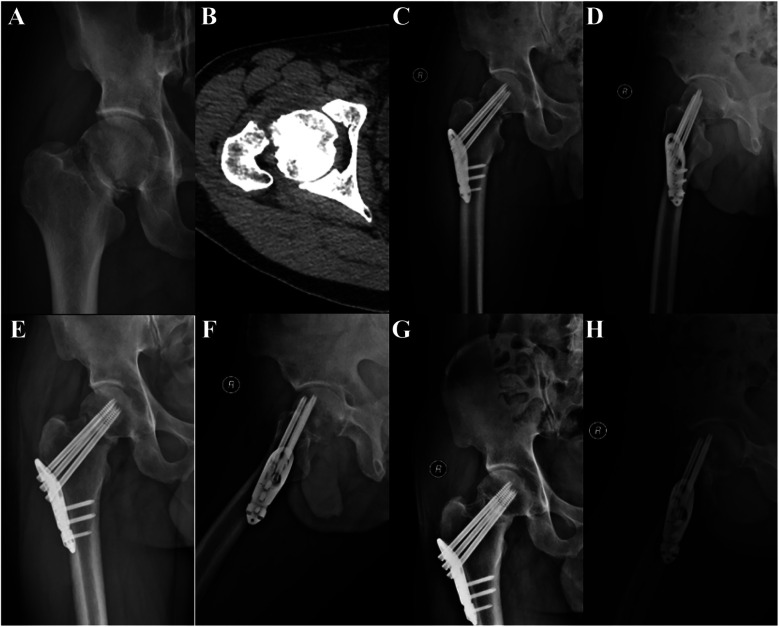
A 52-year-old male patient was admitted to the hospital for treatment of right hip pain and limited range of motion following a fall and underwent PFLP treatment. (A,B) Pre-operative CT images of the patient showed a right femoral neck fracture, Garden type IV. (C,D) Review radiographs 1 day post-operatively in the anteroposterior and lateral positions showed good reduction, but a gap was present at the fractured end. (E,F) Review radiographs 3 months post-operatively showed gradual fracture healing and a reduction in the fracture gap. (G,H) Review radiographs 6 months post-operatively showed disappearance of the gap and good fracture healing.
